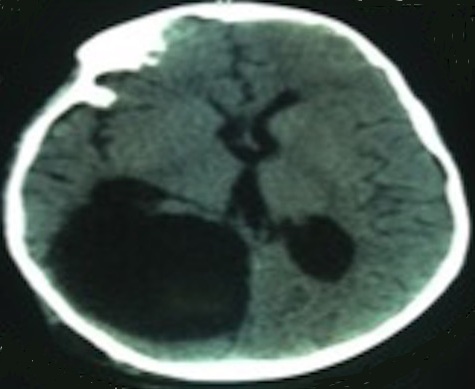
Postoperative CT shows total removal of the lesion
